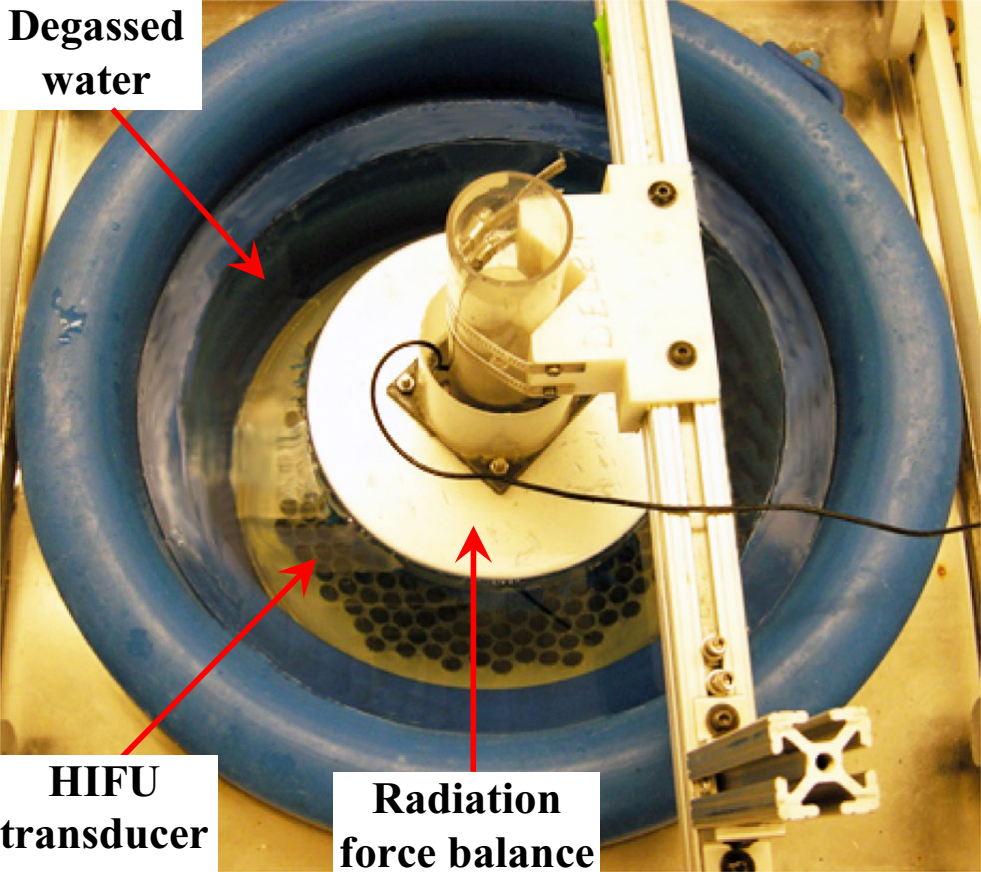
Acoustic power measurement of the lower high‐intensity focused ultrasound transducer by a lab‐built radiation force balance system.
